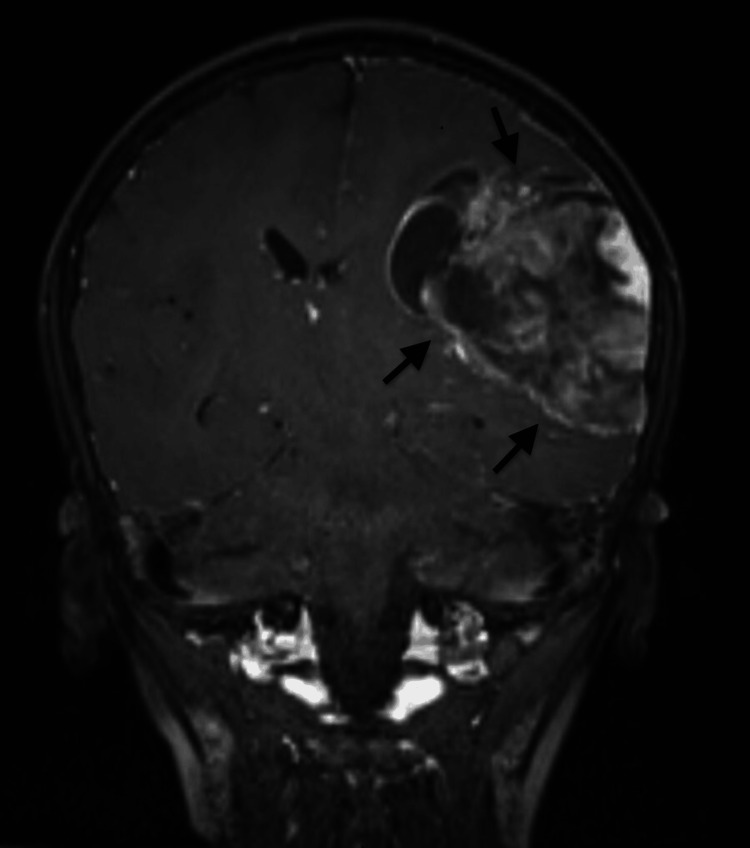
Preoperative magnetic resonance imaging scan in the T1 coronal view Arrows are pointing toward the tumor.
